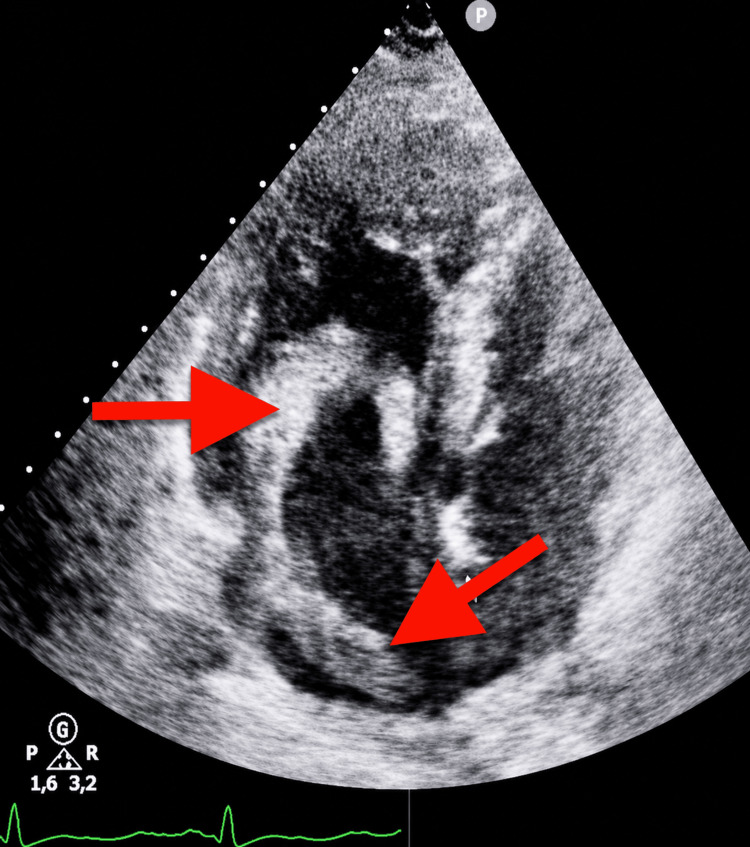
Transthoracic echocardiography, apical four-chamber view: serpentine thrombus visible in the right atrium (red arrows) with prolapse across the tricuspid valve Right ventricular dilation was present, with RV/LV ratio >1 and paradoxical septal motion.
